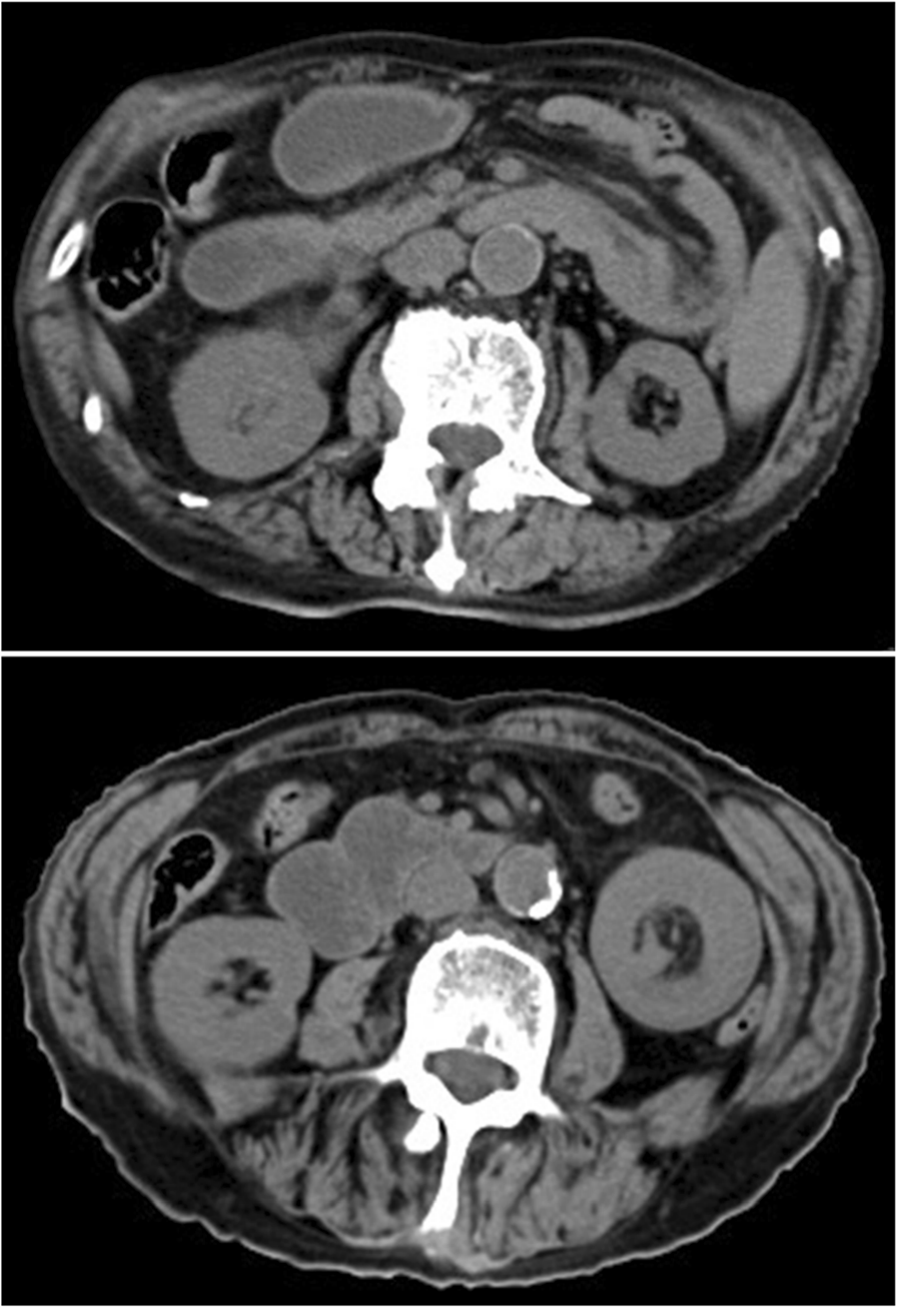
CT shows small bowel dilatation secondary to entero-enteric intussusception
